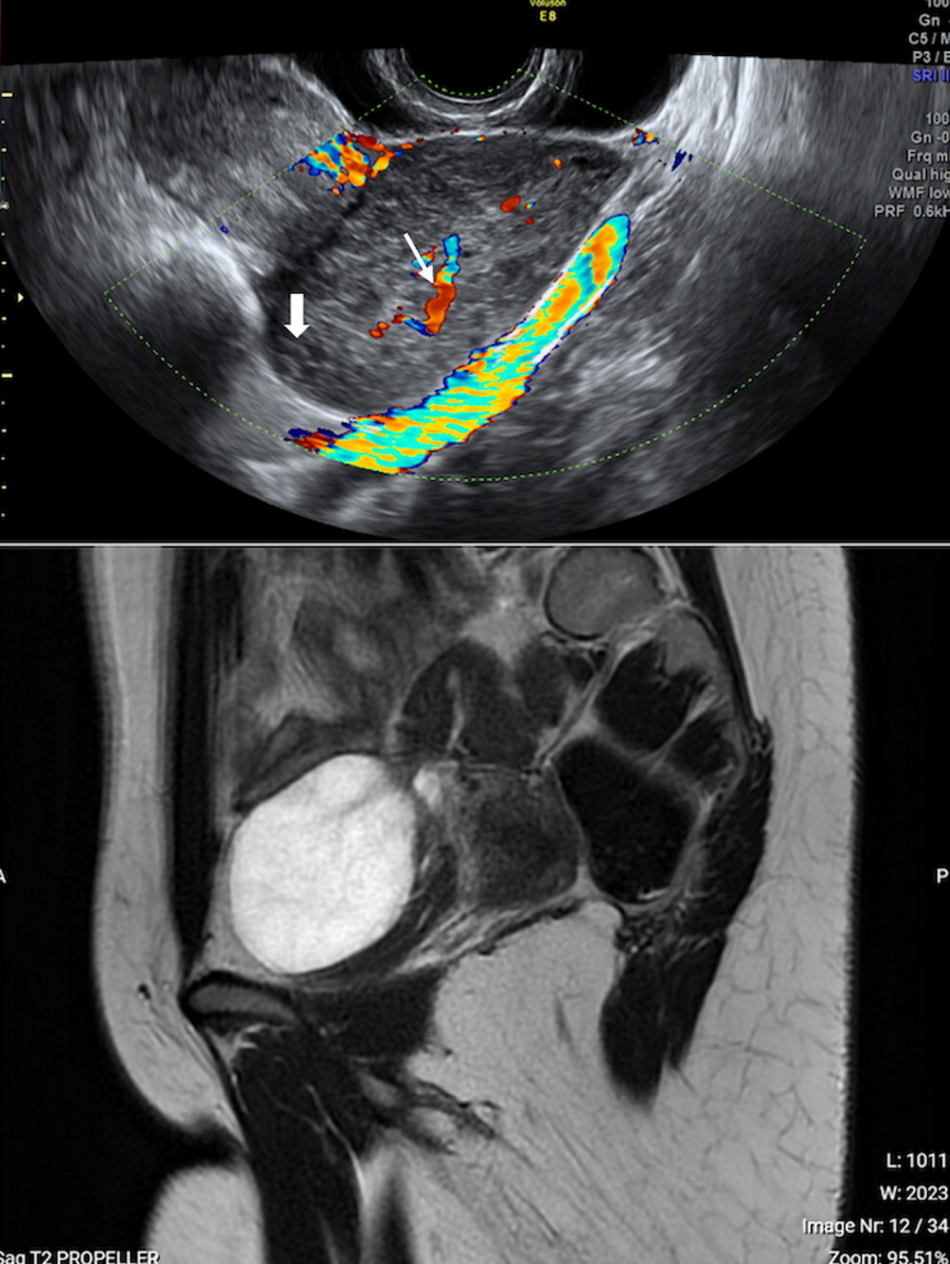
Ultrasound pattern of case 1 (heterogeneous parenchyma without acoustic shadows, with follicles in the periphery, thick arrow, and a central vascular axis color score 2, thin arrow) and corresponding magnetic resonance imaging.
